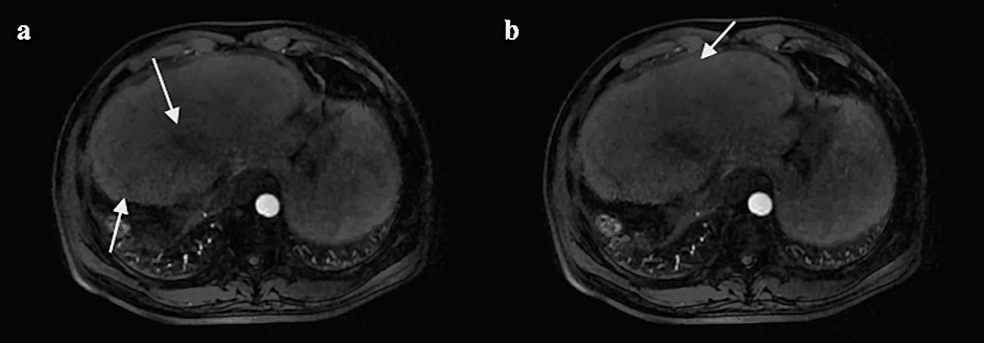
Arterial phase contrast-enhanced MRI nine months after proton beam therapy. (a) The tumor in S4 became invisible and one of the tumors in S2 became a low-intensity signal area. (b) The other tumor in S2 also disappeared.
